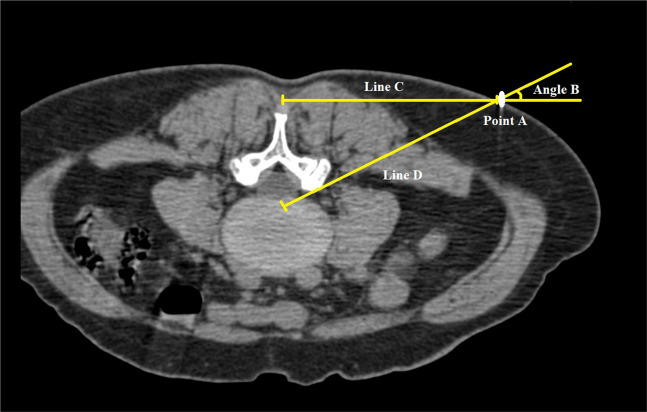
Radiographic evaluation of the puncture trajectory under CT guidance. Point A: Entry point; Angle B: Puncture angle; Line C: Lateral distance; Line D: Puncture trajectory
